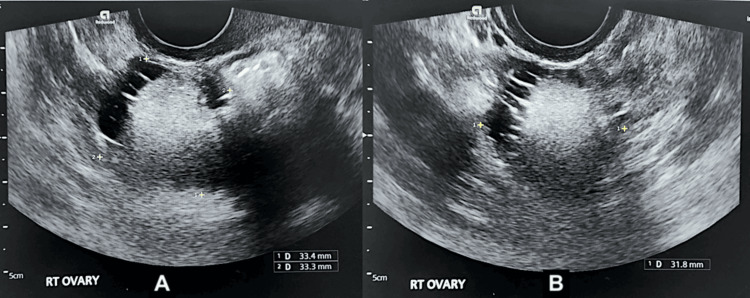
Transvaginal ultrasound of the right ovary demonstrating a dermoid cyst The right ovary (A, B) demonstrating a cyst measuring 33 × 33 × 32 mm. The lesion is predominantly solid with a small fluid-filled area and thin striations, with no internal vascularity on colour Doppler. Features are consistent with a dermoid cyst.
